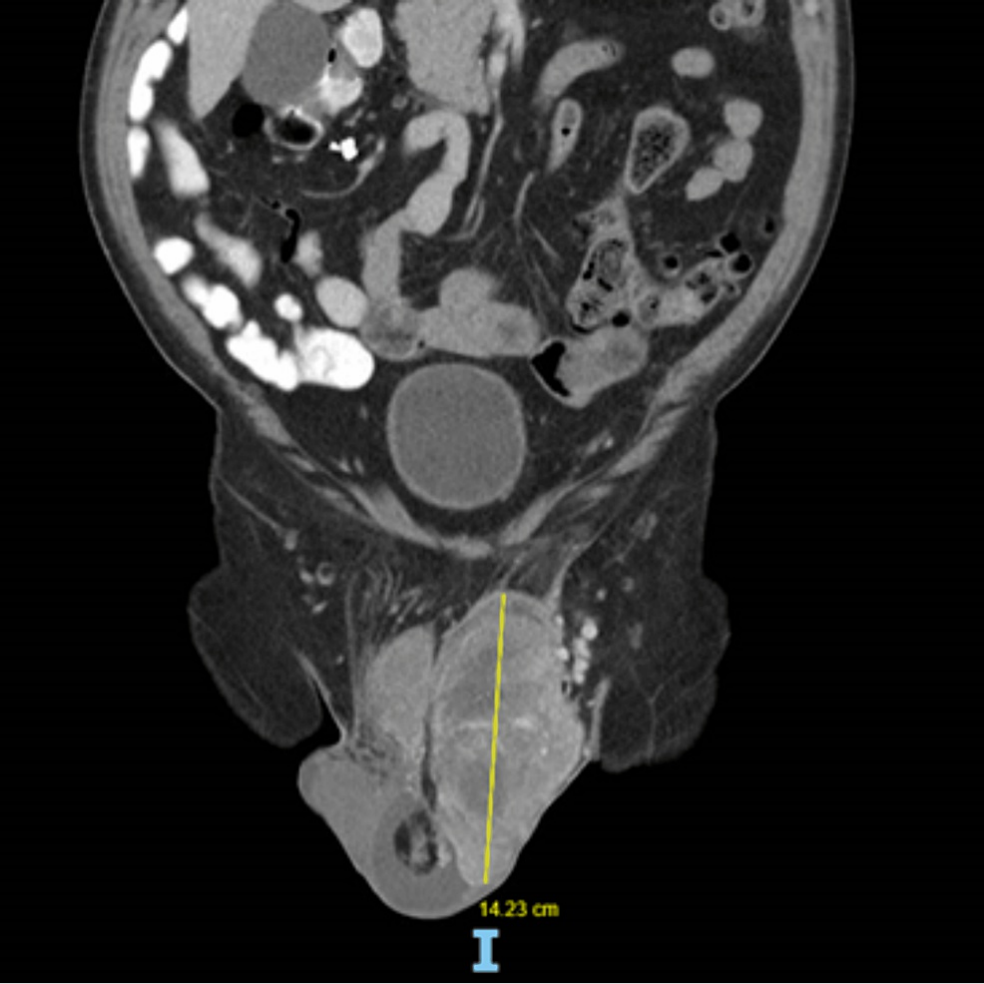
CT abdominal-pelvis scan The image is showing left paratesticular liposarcoma measuring ~14cm.
